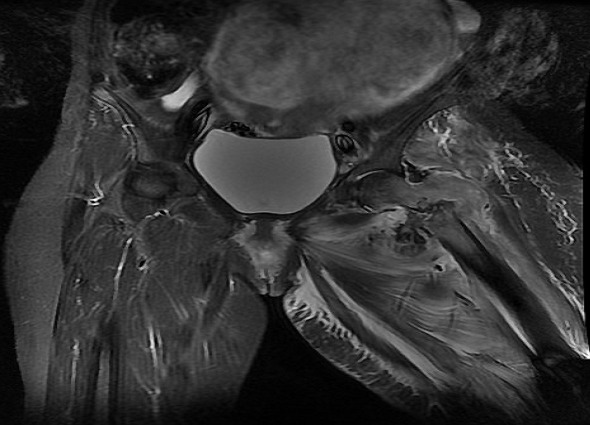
MRI of the thigh, coronal T2 showing a tissular process centred in the left trochanteric region in hypersignal associated with a pathological fracture
